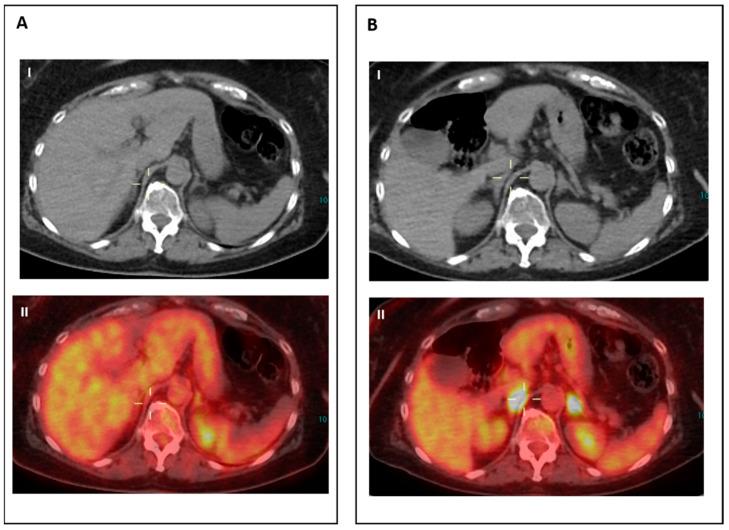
Adrenalitis: patient with advanced melanoma. Panel (A) shows CT (I) and PET/CT (II) during therapy with tyrosine kinase inhibitors; adrenal glands appear normal. Note the appearance of intense hypermetabolism in the adrenal glands (box (B), II) accompanied by symmetrical enlargement in CT images (box (B), I) during combined nivolumab plus ipilimumab treatment.
